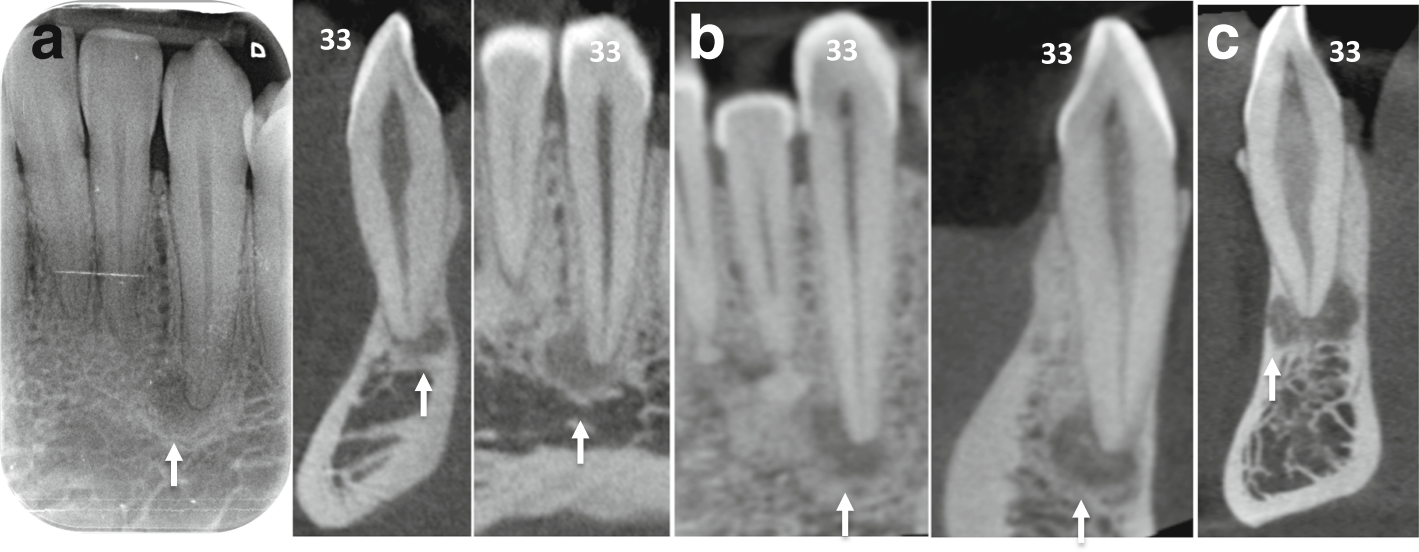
Additional examples of stage 1 OD mimicking endodontic periapical lesions. Careful examination of stage 1 OD depicted in CBCT might indicate faint differences as compared to endodontic lesions. For instance, the periapical OD radiolucency could appear slightly more dense compared to the surrounding trabecular space (*white arrows* in a) and/or can include discrete central hyperdense structures surrounded by a hypodense margin (*white arrows* in b). Moreover this complex is separated from the regular cancellous bone by a thicker hyperdense line. The whole process can protrude into the compact bone as well (*white arrows* in c)
